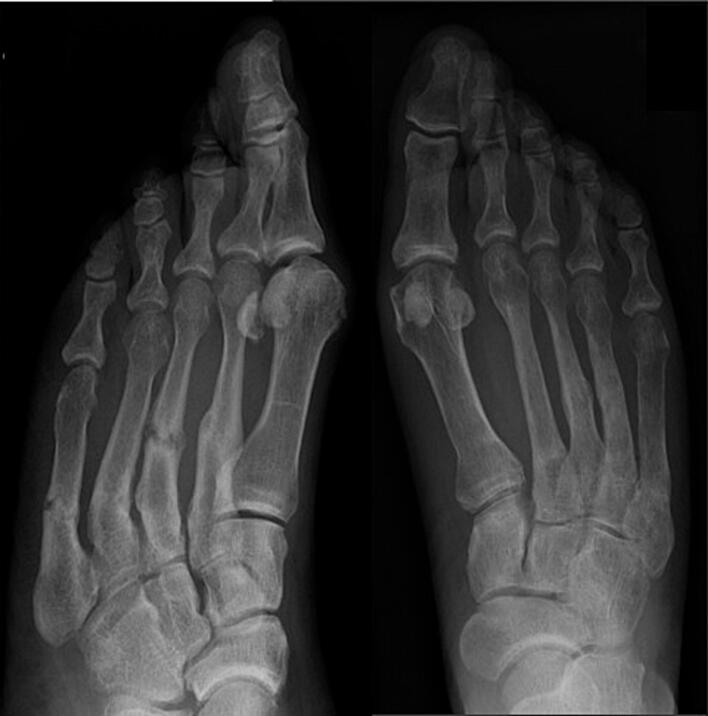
Radiographic image of metatarsal bone fractures II-V bilaterally.
